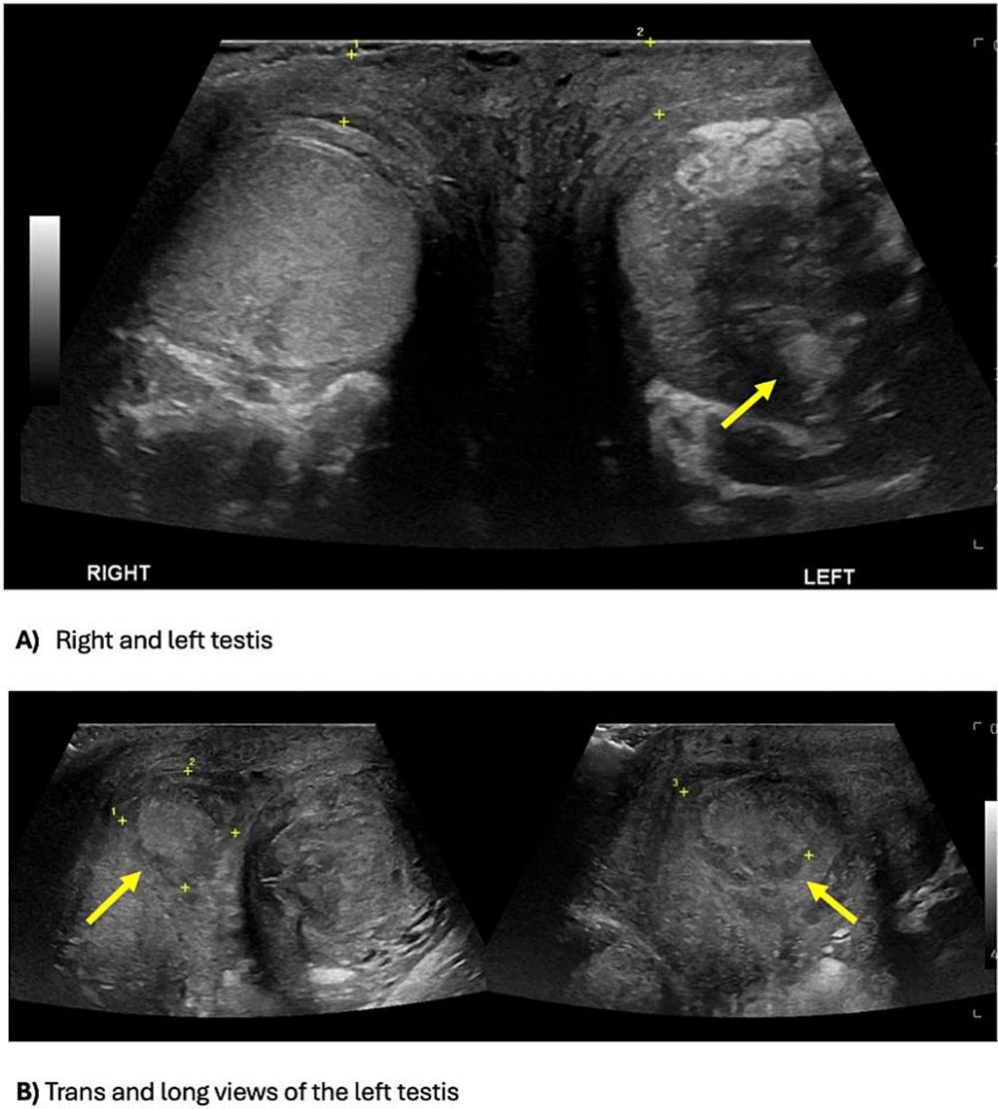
Scrotal ultrasound demonstrating an ill-defined and heterogeneous left testis with a central hypoechoic part of decreased vascularity suggestive of an abscess, versus the homogenous and normal vascularity right testis (A). The testicular abscess extends medially through the tunica albuginea, causing a scrotal wall abscess (B).
